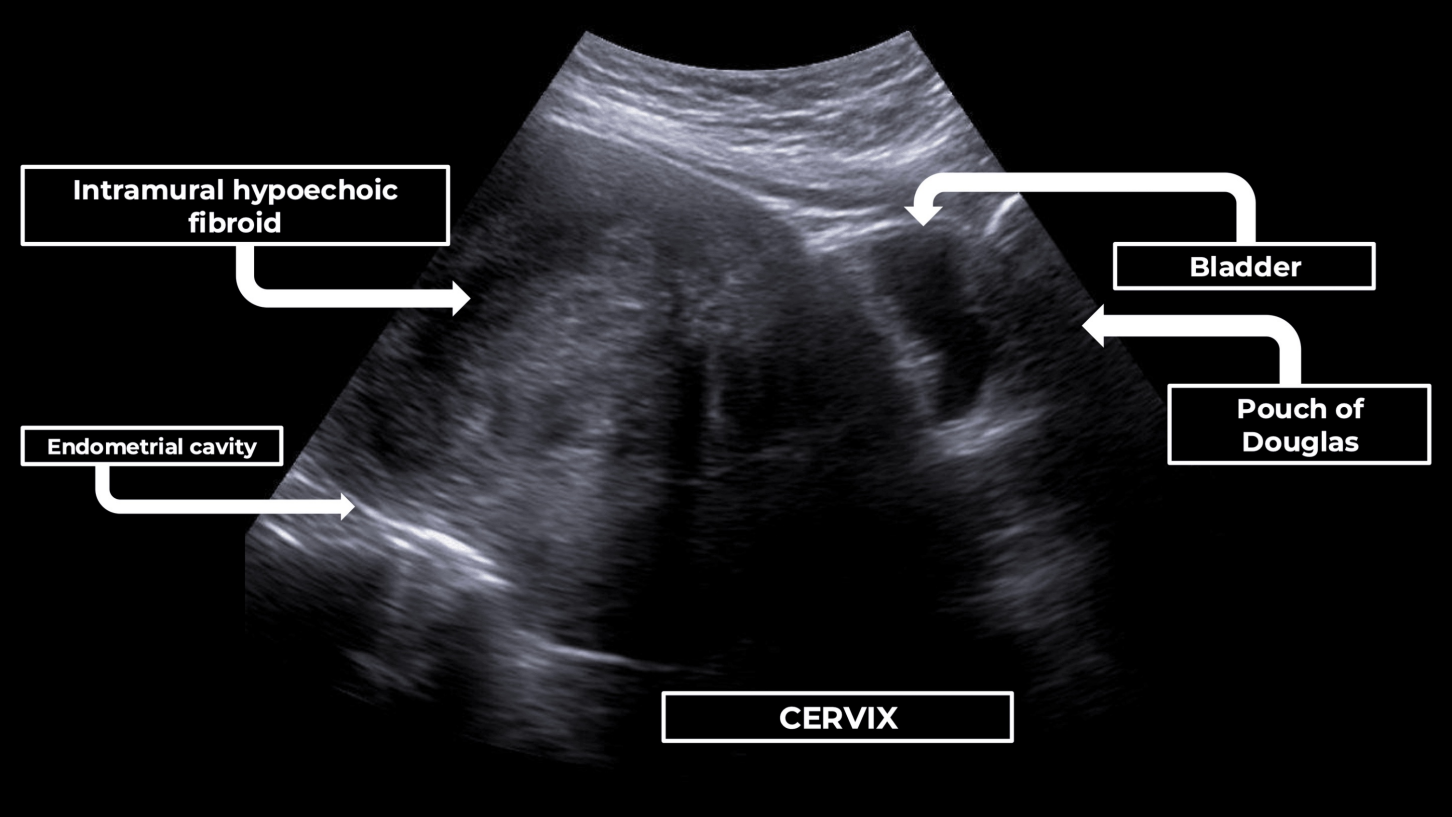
Pelvic ultrasound Showing a hypoechoic intramural uterine fibroid (FIGO type 4–5) and normal anatomic orientation of the bladder, cervix, and pouch of Douglas. According to the FIGO classification system (PALM-COEIN) for abnormal uterine bleeding (Munro et al., 2011 [9]). FIGO: International Federation of Gynecology and Obstetrics; PALM-COEIN: Polyp, Adenomyosis, Leiomyoma, Malignancy, Coagulopathy, Ovulatory Dysfunction, Endometrial, Iatrogenic, and Not-yet-classified
